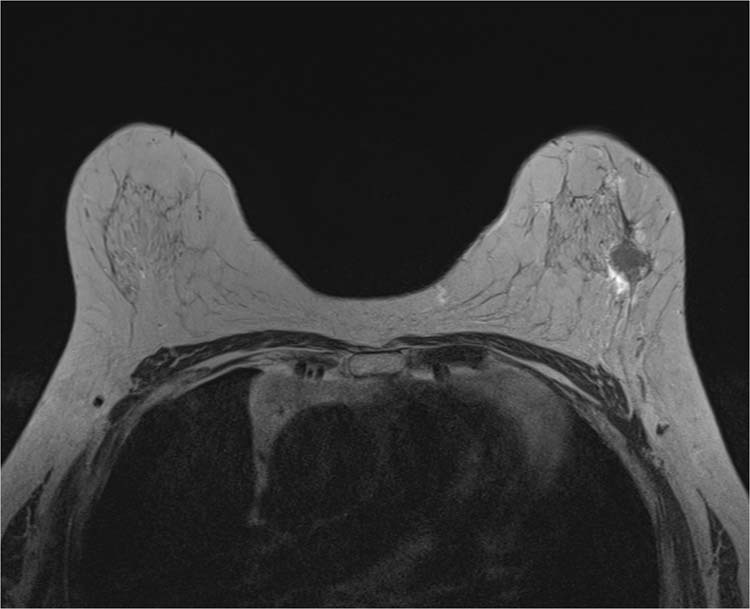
On axial T2-weighted image, high signal intensity which is defined as peritumoral edema, is noted at posterior aspect of breast tumor in left upper outer breast.
